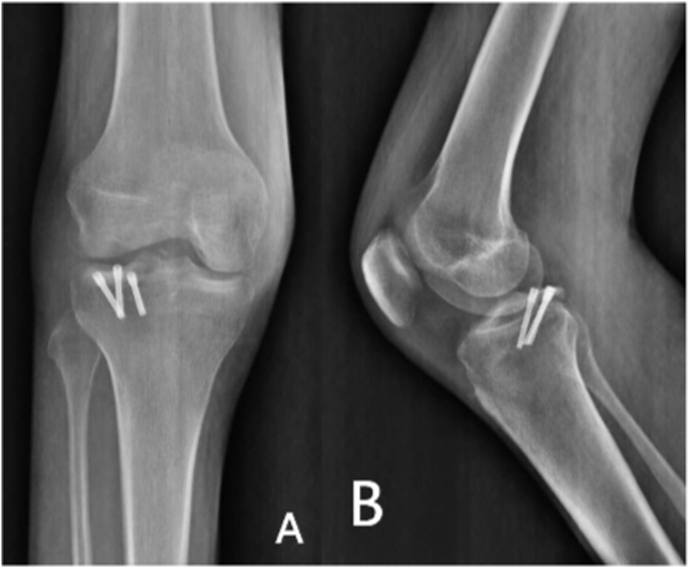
Anteroposterior (A) and lateral (B) X-rays; Three weeks after the surgery after removing the cast.
